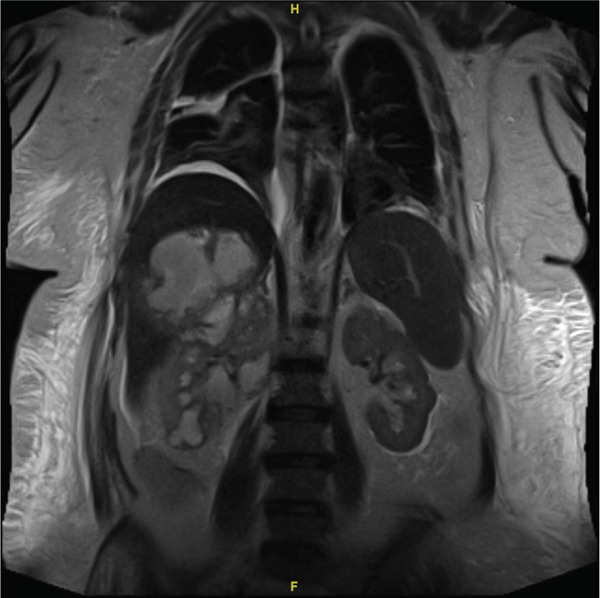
Magnetic resonance imaging performed 7 days after initial presentation demonstrates right hydronephrosis secondary to an obstructing ureteropelvic junction (UPJ) stone. A large, complex intrarenal abscess is present in the upper pole of the right kidney, measuring 5.7 × 5.9 × 6.2 cm, with extension into the perinephric space and direct abutment of a second multiloculated collection in the right hepatic lobe measuring 7 × 7.6 × 6 cm. These findings raise concern for extension of the perinephric abscess into the liver; however, a definitive fistulous tract is not visualized on this study.
